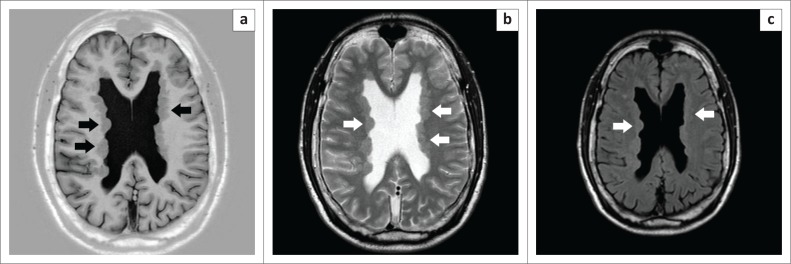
MRI scan sections of the patient’s brain indicating the presence of GMH. (a) A T1W IR SENSE axial section of the brain with arrows indicating bilateral and asymmetrical PNH with heterotopic grey matter stretching all along the ventricular walls, even present anterior to the anterior horns. Note that the shade of grey is the same as that of the cortical grey matter (the same signal intensity), which confirms that it is grey matter – the pathognomonic finding in GMH. (b) The arrows point towards the PNH where the typical nodular appearance of the heterotopic grey matter can be seen on this T2W TSE SENSE axial section of the brain. (c) On this T2W CLEAR SENSE GAD enhanced axial section of the brain, one can see how the subependymal nodules cause bulging of the ventricular walls, as pointed out by the arrows. GMH – grey matter heterotopia; T1W – Tau 1 weighted; T2W – Tau 2 weighted; FLAIR – fluid-attenuated inversion recovery; IR – inversion recovery; FFE – fast field echo; SENSE – sensitivity encoding; TSE – turbo spin echo; CLEAR – constant level appearance; GAD – gadolinium; PNH – periventricular nodular heterotopia.
